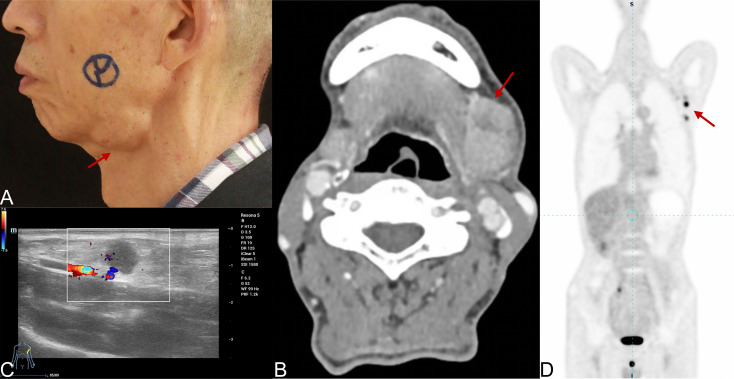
Preoperative clinical and imaging findings of submandibular salivary duct carcinoma with axillary lymph node metastasis. (A) Clinical photograph showing a firm mass in the left submandibular region (red arrow). (B) Contrast-enhanced CT image revealing an irregular soft-tissue mass with heterogeneous enhancement and central necrosis (red arrow). (C) Color Doppler ultrasonography of the left axilla demonstrating a rounded hypoechoic lymph node with blurred cortical–medullary differentiation and increased vascularity (red arrow). (D) PET/CT scan showing multiple FDG-avid lesions in the left axillary region (red arrow), indicating metastatic lymphadenopathy.
